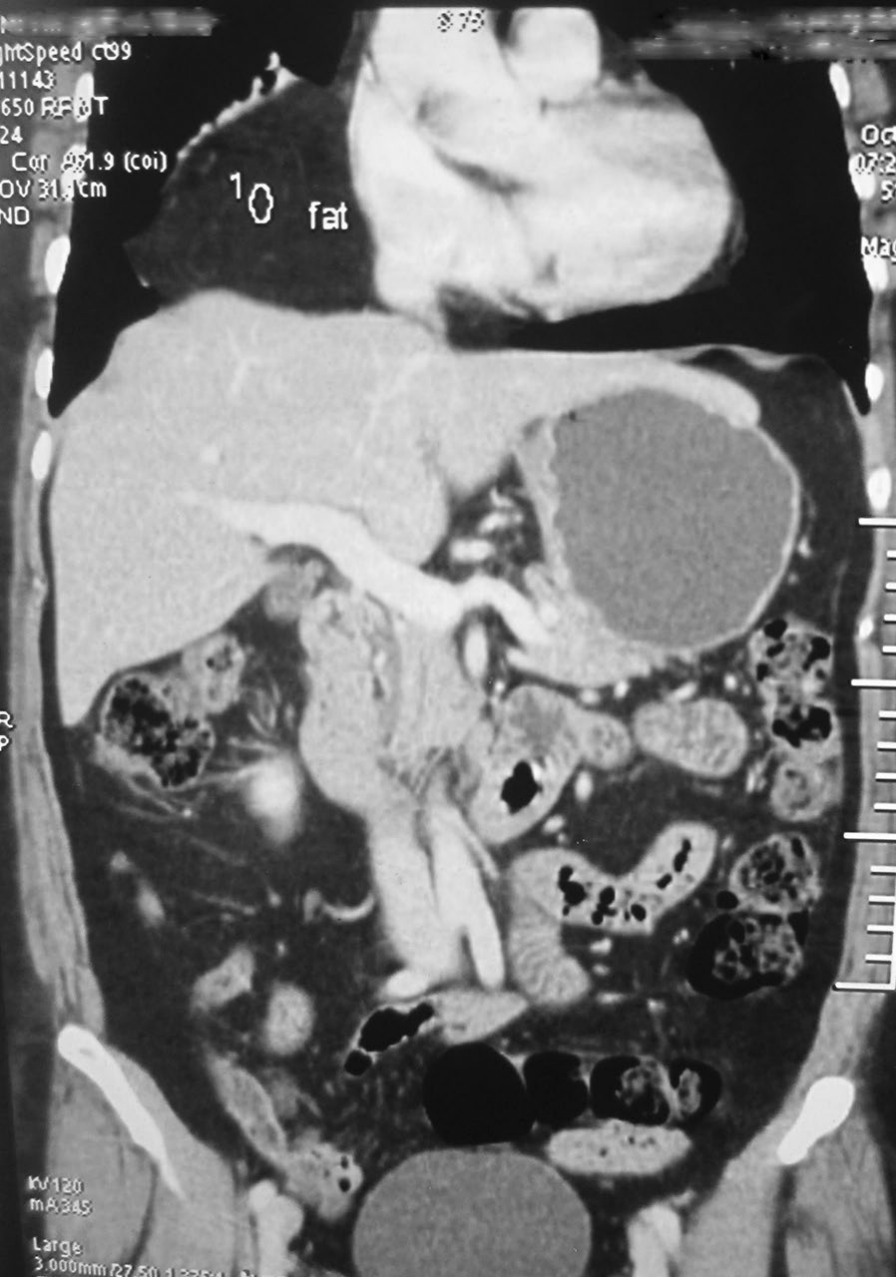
Preoperative computed tomography image of the thorax and abdomen in patient 2 showing Morgagni hernia.
